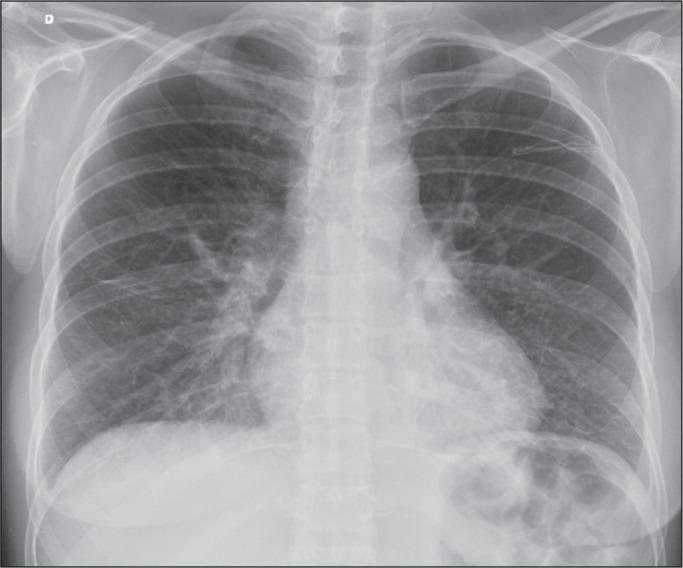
A 32-year-old patient hospitalized for a pulmonary infection. Chest X-ray
